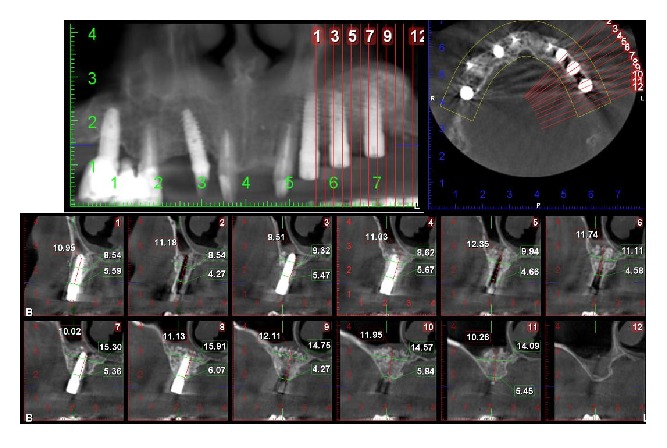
CBCT showing the implants surrounded by dense tissue six months postoperatively.
